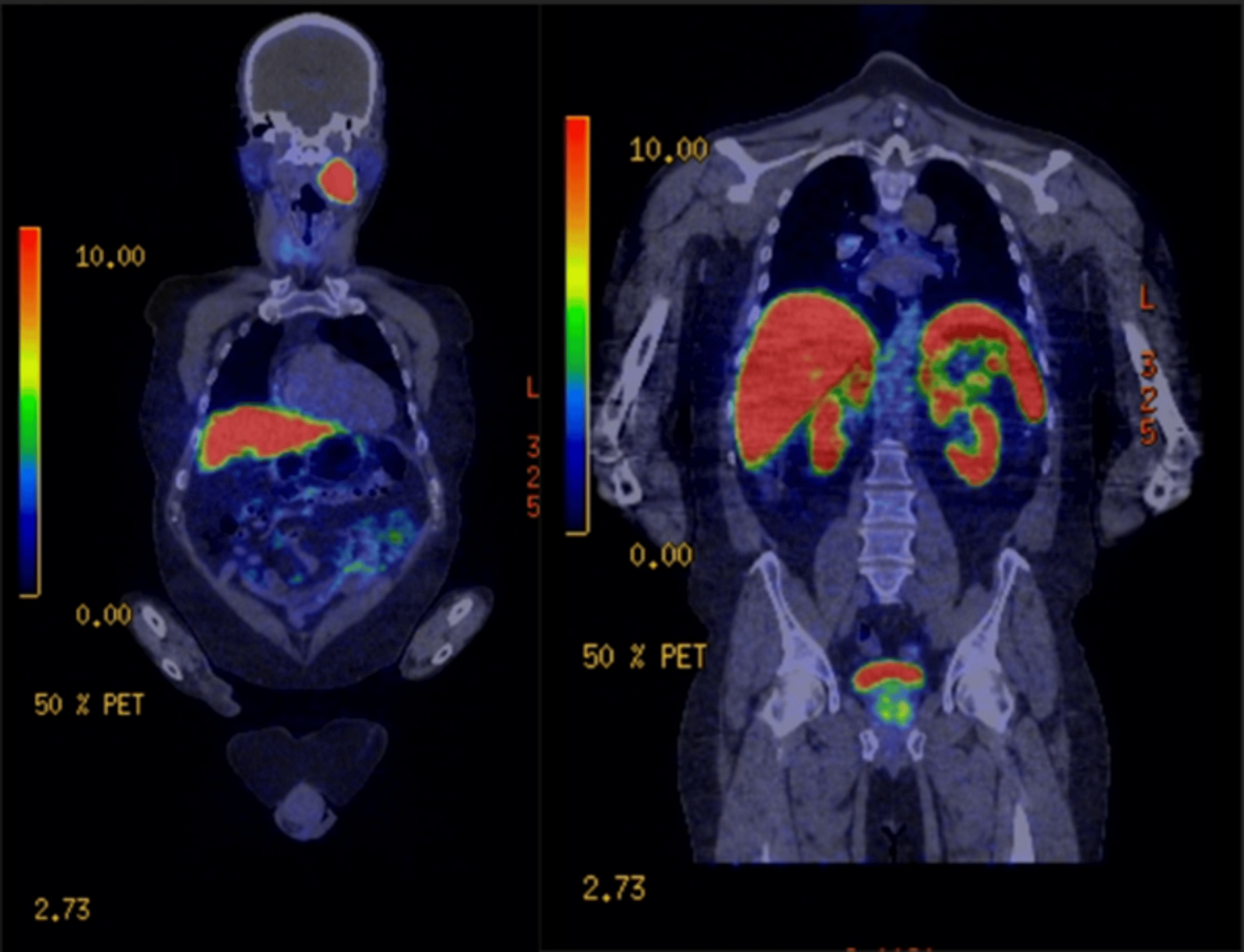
DOTA-TATE PET-CT showing increased uptake in the left neck (left panel), but no increased uptake in the retroperitoneal para-aortal area (right panel)
